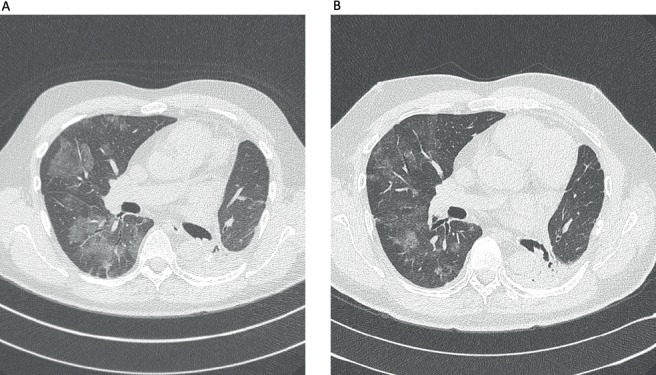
Chest CT findings before and after corticotherapy. (A): Patchy ground-glass opacity, mainly in the right lung. (B): Significant improvement after 3 weeks with prednisone.
